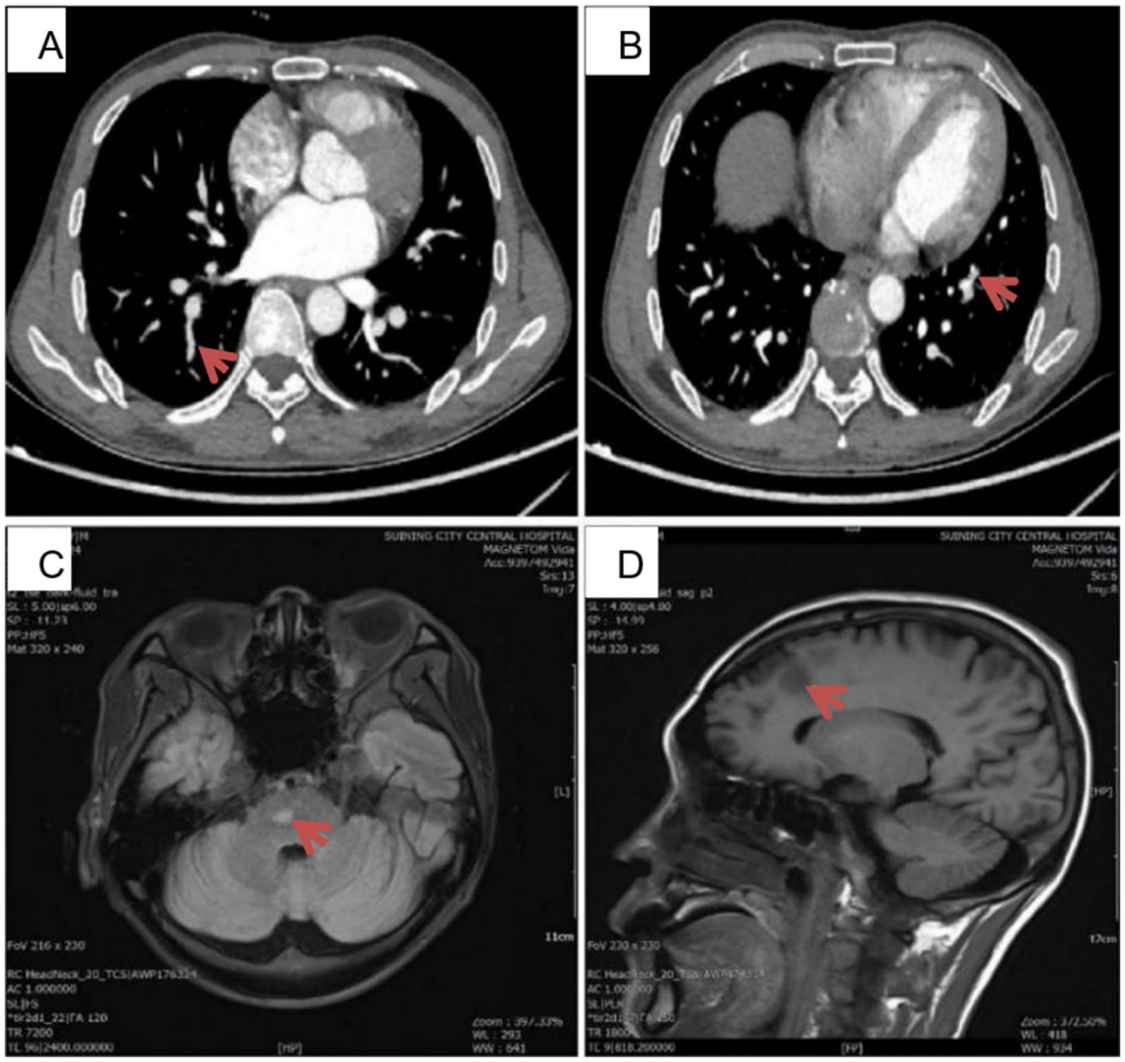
(A,B) The patient’s pulmonary artery CTA images on February 20, 2024. In the lower lobes of both lungs, multiple pulmonary arteries are not clearly shown, and filling defect areas can be seen. The posterior basal segment artery of the right lower lobe is shown in (A) (red arrow). The anterior medial basal segment artery of the left lower lobe is shown in (B) (red arrow), with filling defects. (C,D) The plain and enhanced MRI scans of the head and whole spinal cord taken in our hospital on February 29^th^, 2024. There are newly emerged abnormal enhancement foci in the right frontal lobe, the centrum semiovale, and the pons. The red arrow in (C) shows the patient’s abnormal lesion at the pons level. The red arrow in (D) shows the patient’s abnormal lesion at the right frontoparietal lobe level.
